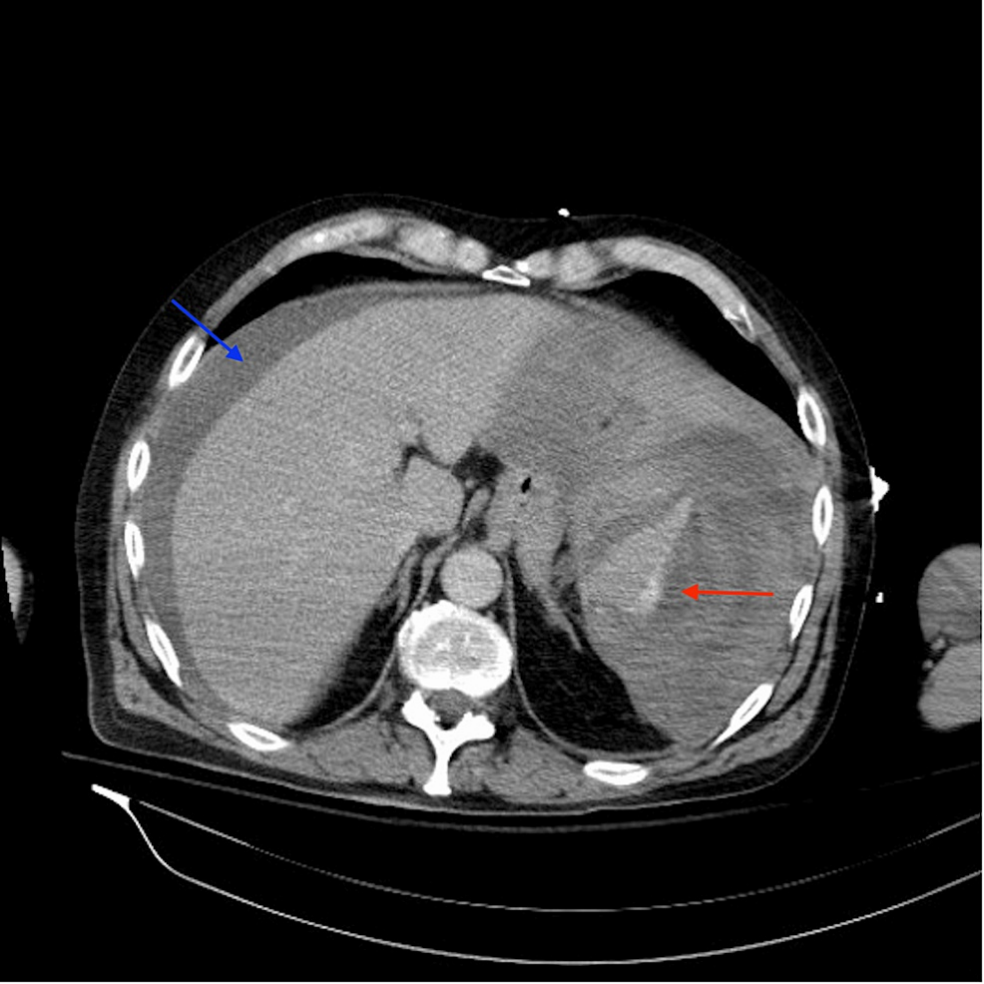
CT abdomen with contrast CT abdomen with contrast revealing a large subcapsular hematoma compressing the spleen (red arrow), with mild-to-moderate serosanguineous ascites (blue arrow). Subcapsular venous extravasation is noted under the lateral splenic capsule.
